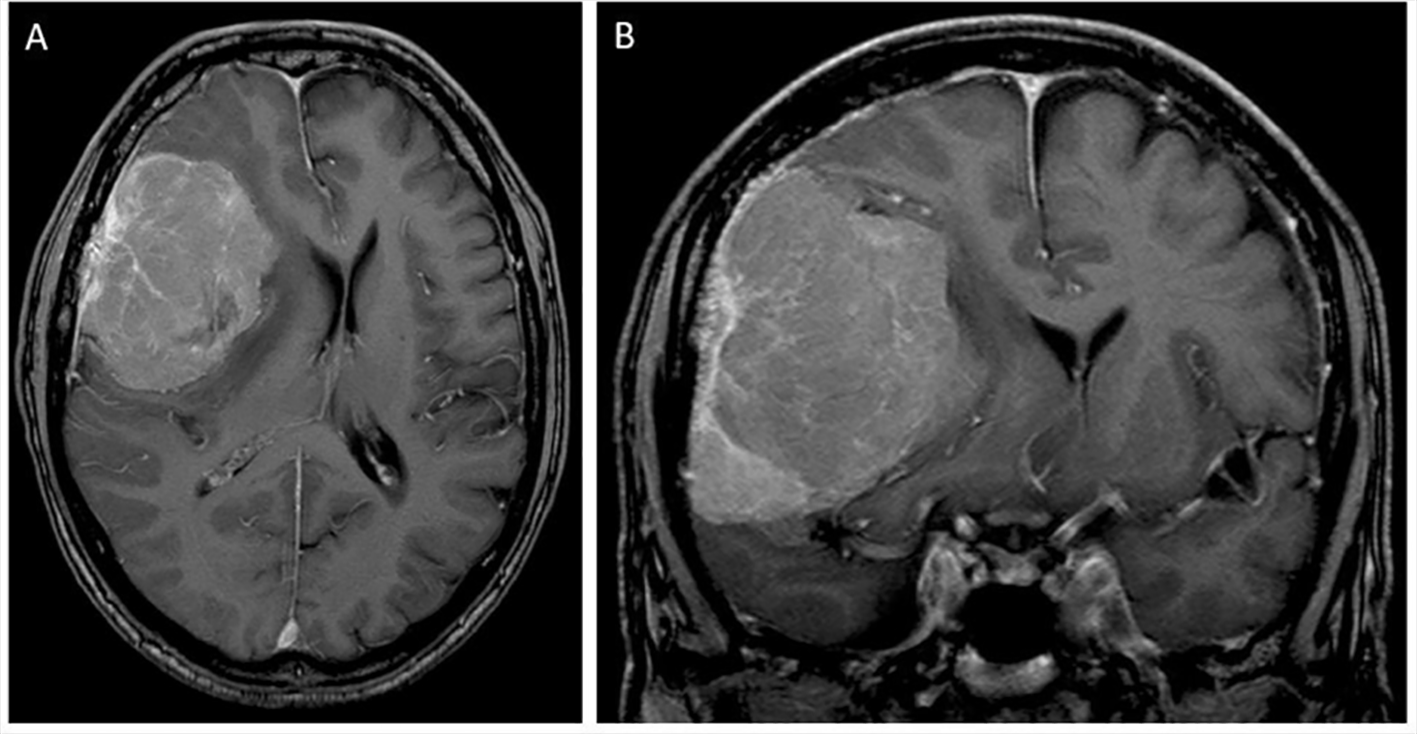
T1-contrast-enhanced MRI scan in axial (A) and coronal (B) sections at the initial diagnosis showing a large right frontal (approximately 5.5 cm × 7.0 cm) tumor with dural attachment, suggestive of a meningioma, with midline and brain shift.
